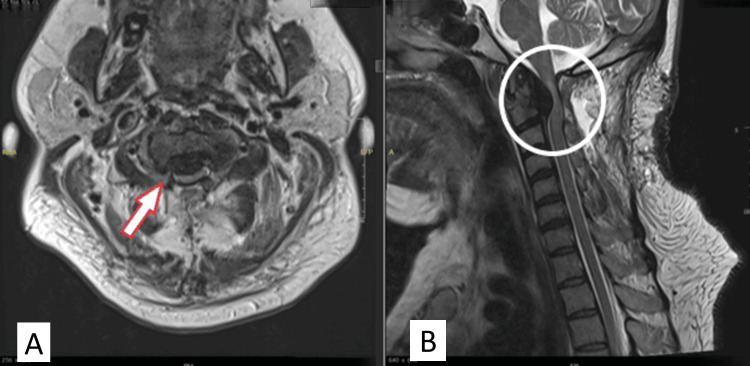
Post-operative cervical MRI. The axial view (A) showed significant cord compression by a retro-odontoid mass (arrow). The sagittal view (B) showed an area of posterior decompression with mild improvement of cord compression in comparison to the initial MRI and abnormal hypersignal intensity of the spinal cord (circle).
